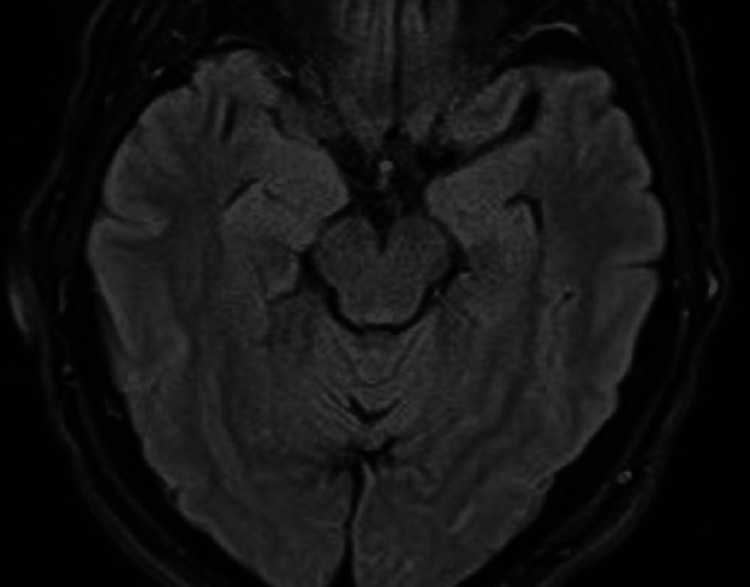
MRI fluid-attenuated inversion recovery (FLAIR) sequence eight months after the episode
